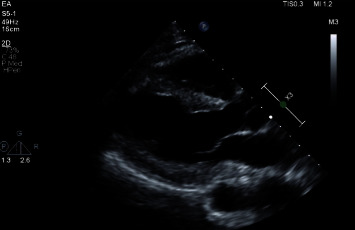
Transthoracic Echocardiogram 4 weeks after initial presentation, showing trace circumferential pericardial effusion. The maximum diastolic dimension of the pericardial effusion seen is 7 mm posteriorly. There are no echocardiographic findings for cardiac tamponade. TEE at a 2-monthfollow-up showed no significant changes.
